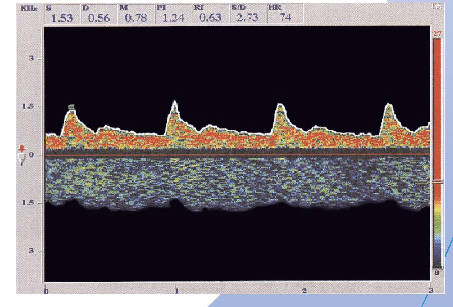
Clinical interpretation of the functional state of brain arteries and veins through ultrasound dopplerography. Only methods of clinical interpretation of the functional state of arteries and veins in the brain with the help of ultrasound dopplerography can localize signs of the arteriovenous shunting and define its size and risk of development of deficit in regional blood supply [25-27,30].
